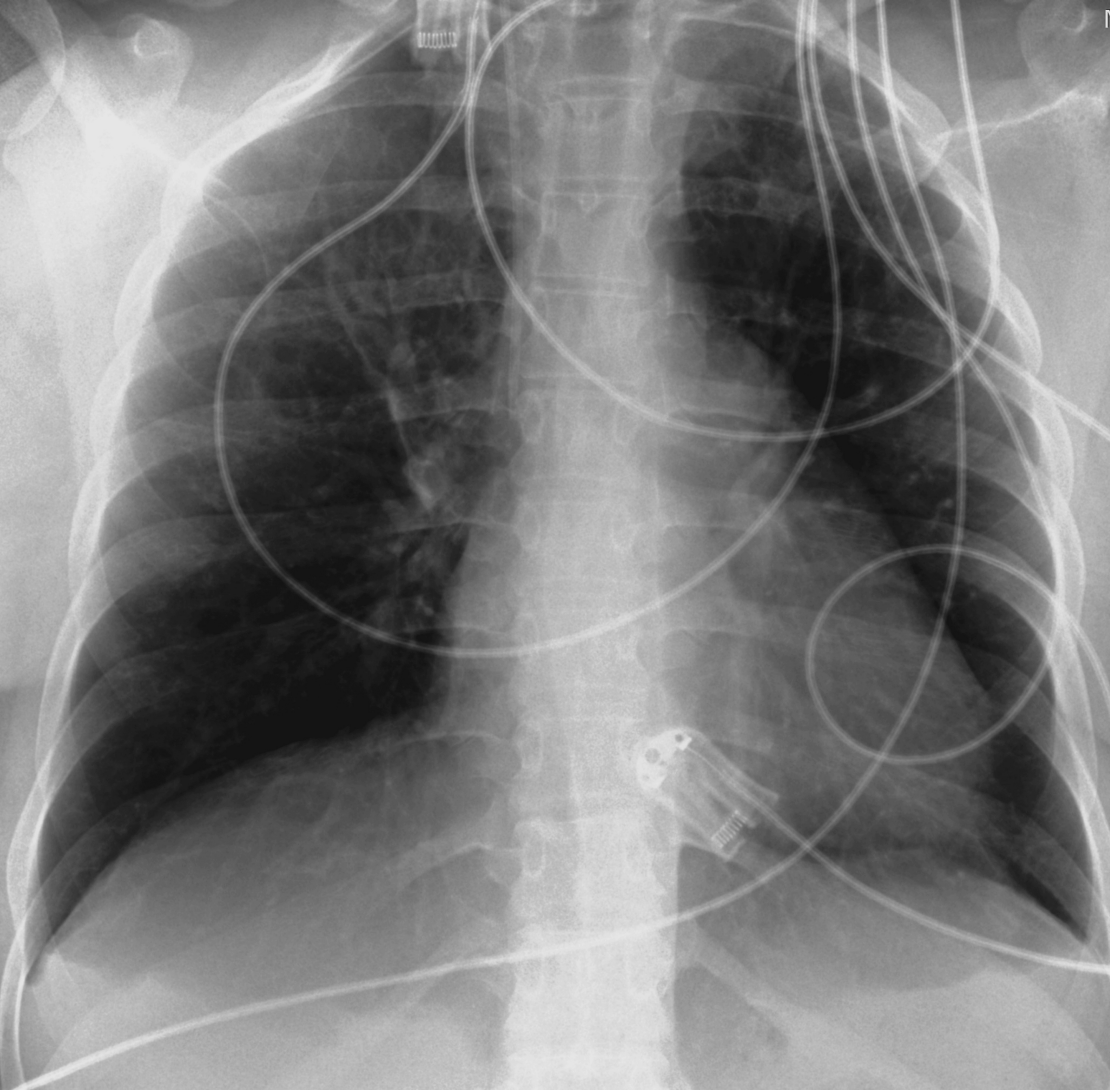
X-ray of chest on the day of discharge from the ICU (Intensive Care Unit) which was normal.
